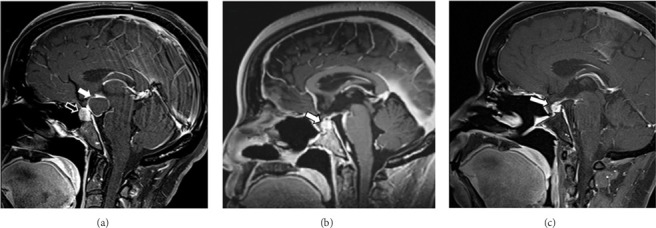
MRIs: (a) preoperative MRI: MRI postcontrast demonstrating a heterogeneously enhancing lesion seen in the left sellar measuring 1 cm × 9 mm × 1 cm (open arrow). There is a contiguous suprasellar mass which is predominantly cystic measuring 2.9 × 2.8 × 2.7 cm (solid white arrow). (b) Postoperative MRI showed a residual enhancing lesion in the sella and along the infundibulum (arrow). There is a decreased bulk of residual enhancing tissue in the sella compared to preoperative imaging. This demonstrates slightly decreased T1 hyperintense signal abnormality/enhancement in the right suprasellar region extending towards the interpeduncular cistern. (c) 6 months follow-up MRI showed the residual enhancing lesion in the sella decreased from the previous study (arrow).
